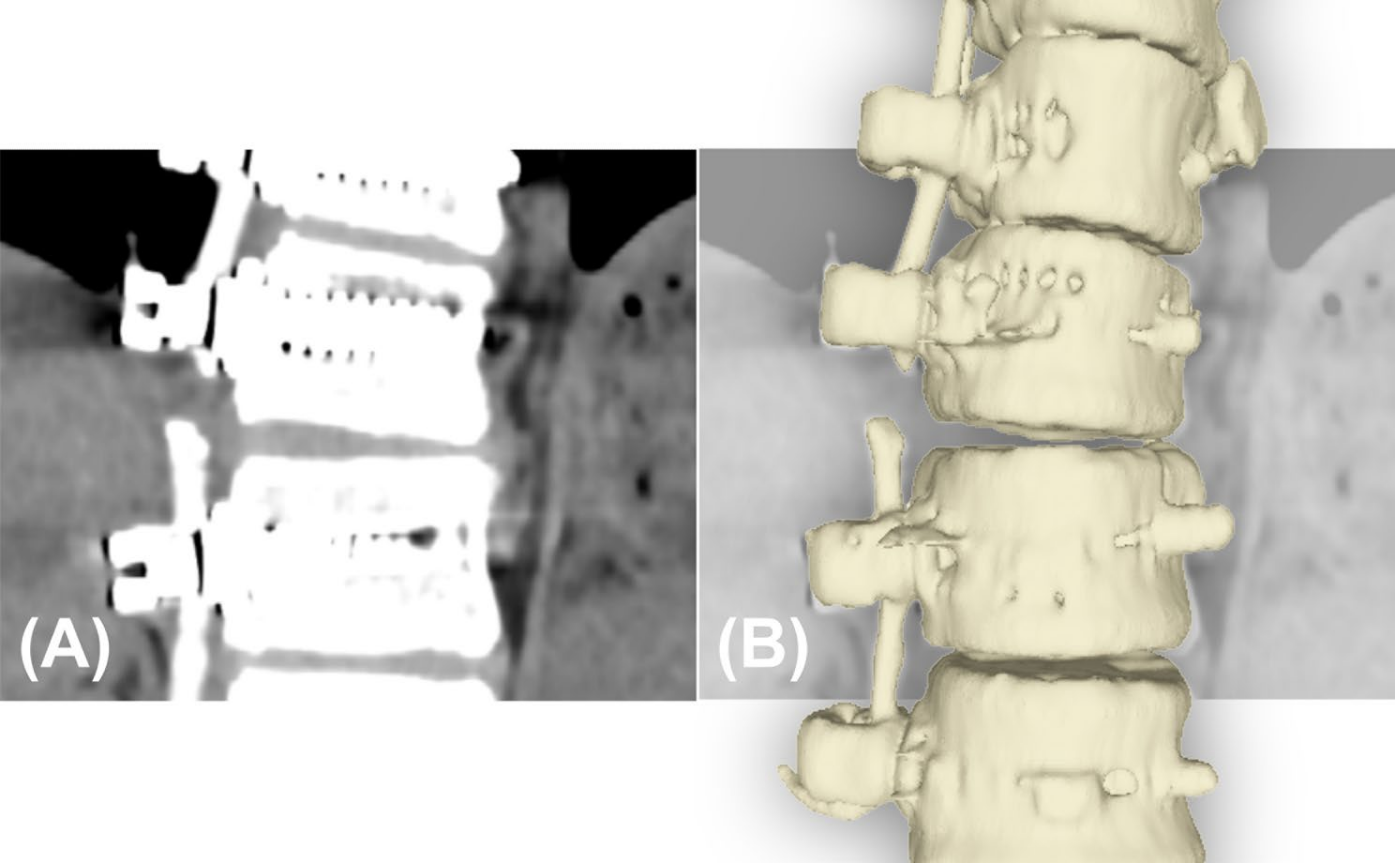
Example tether breakage located at T11–T12 screw–tether junction where the discontinuity between the tether segments on 2D CT slice is clearly visible (A), and its 3D reconstruction for visualization purposes (B)
